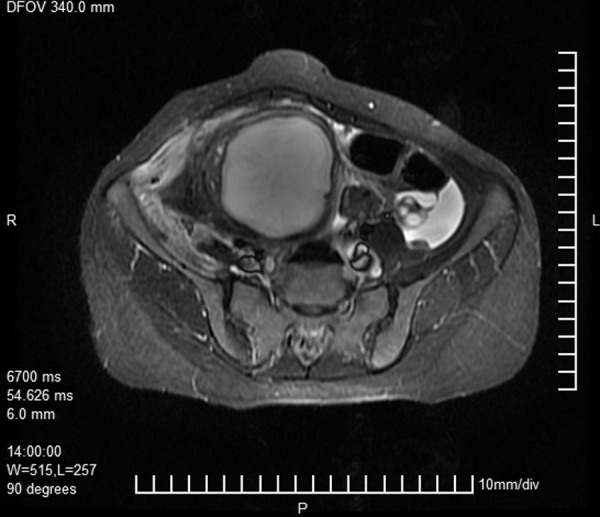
Axial T2W MRI image showing large hyperintense right adnexal
